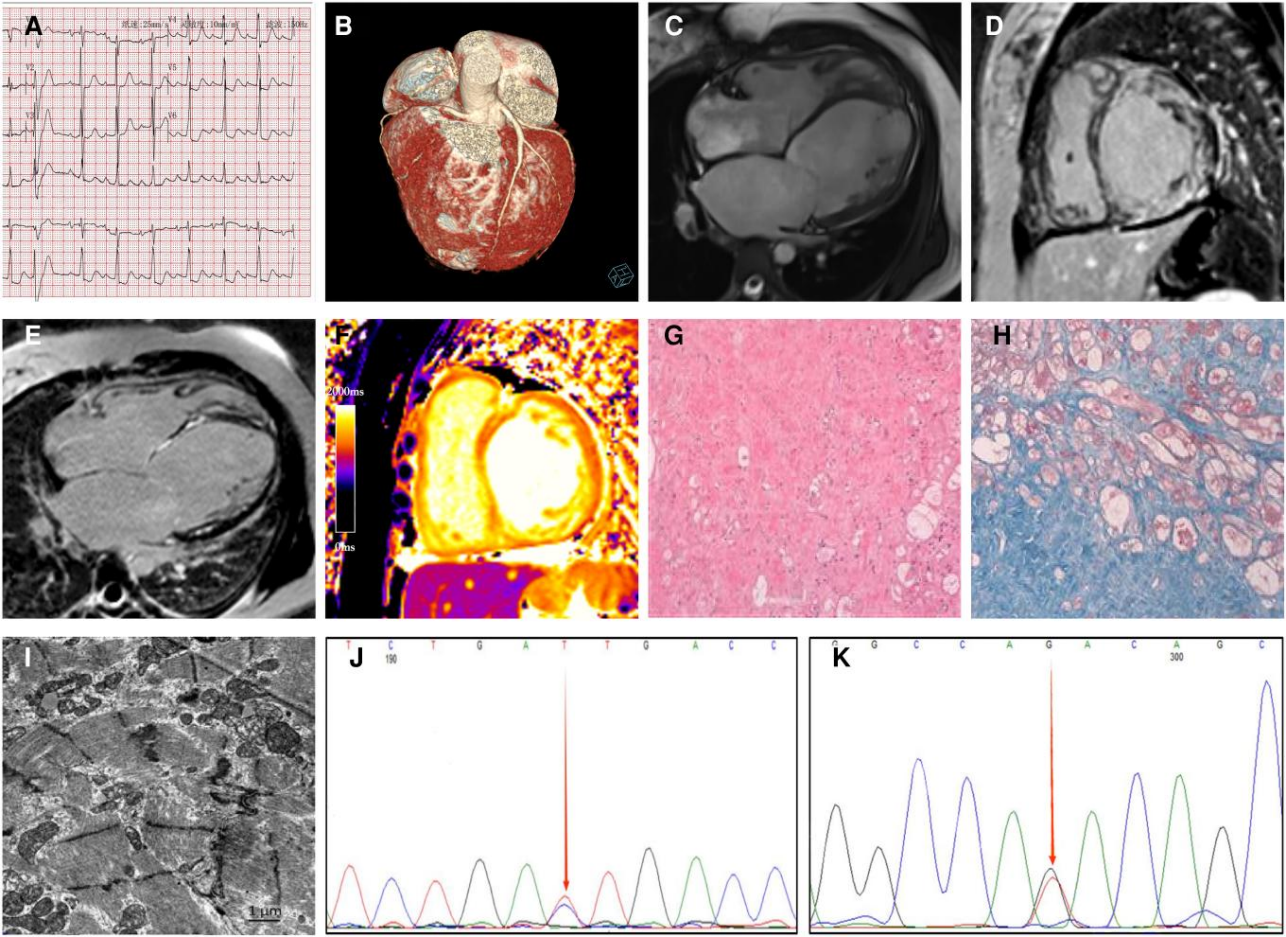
Transthoracic echocardiography at admission demonstrated global cardiac enlargement with diffuse left ventricular hypokinesia and markedly reduced systolic function (left ventricular ejection fraction 33%). The interventricular septum and left ventricular wall were diffusely thinned, with reduced wall thickening throughout the cardiac cycle. Left ventricular end-diastolic diameter measured 61 mm. No focal regional wall motion abnormalities were identified. Reduced systolic excursion of the mid-to-apical right ventricular free wall was also noted. In addition, moderate mitral regurgitation, mild-to-moderate tricuspid regurgitation, and pulmonary hypertension (mean pulmonary artery pressure > 36 mmHg) were present.
